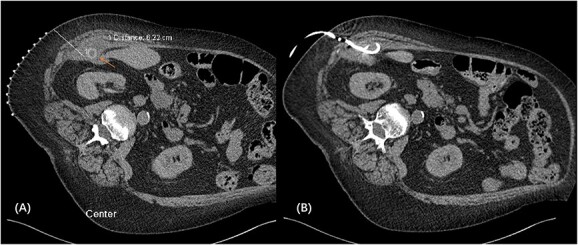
CT-guided pigtail re-insertion pre-operatively to assist localization of gallstone (A); drain placed in the right subhepatic collection located within the transverse abdominis muscle (B).
